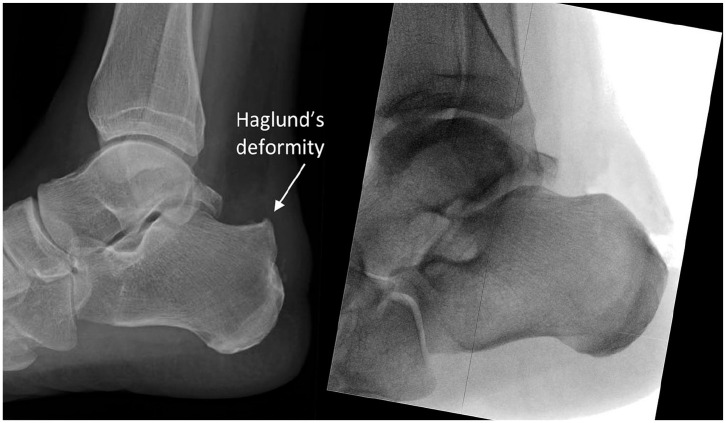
Comparison of preoperative lateral radiograph (left) showing Haglund’s deformity and intraoperative lateral radiograph (right) following bony debridement.
